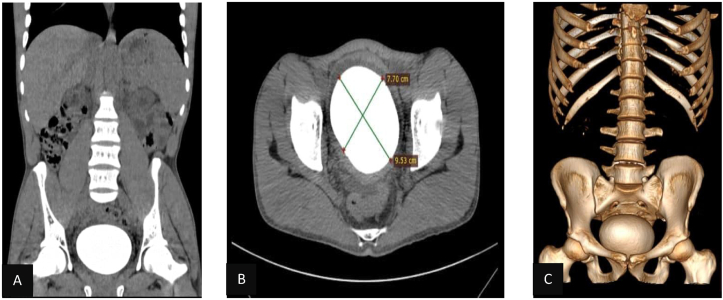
Pre-operative non-contrast CT of the abdomen and pelvis. A: Coronal Reconstruction B: Axial image C: 3D reconstruction.
